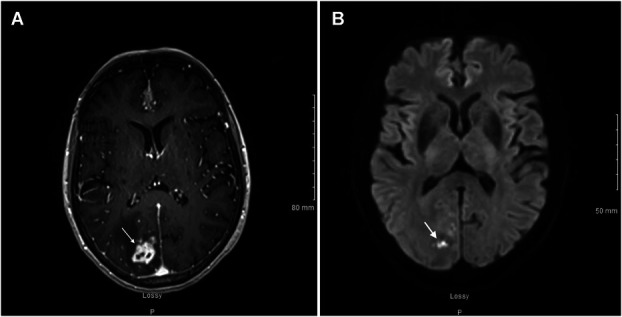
Axial T1 MRI postcontrast A, before abscess resection showing an irregular, enhancing intra-axial mass in the right parasagittal occipital lobe measuring 2.2 × 1.6 × 1.9 cm (white arrow), as well as an axial diffusion-weighted imaging B, showing a few regions of punctate diffusion restriction (white arrow).
